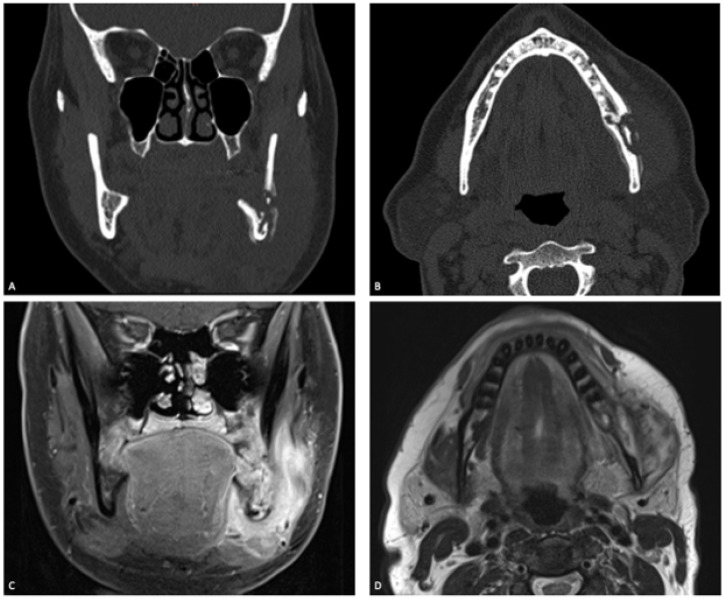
Coronal (A) and axial (B) CT-scans of a 52-year-old woman with secondary chronic osteomyelitis of the mandible. Osteolysis, sequestration, and periosteal reaction were shown on the left side of the mandible. Coronal (C) and axial (D) MRIs showed the soft tissue involvement with signs of myositis of the left masseter and mylohyoid muscles.
